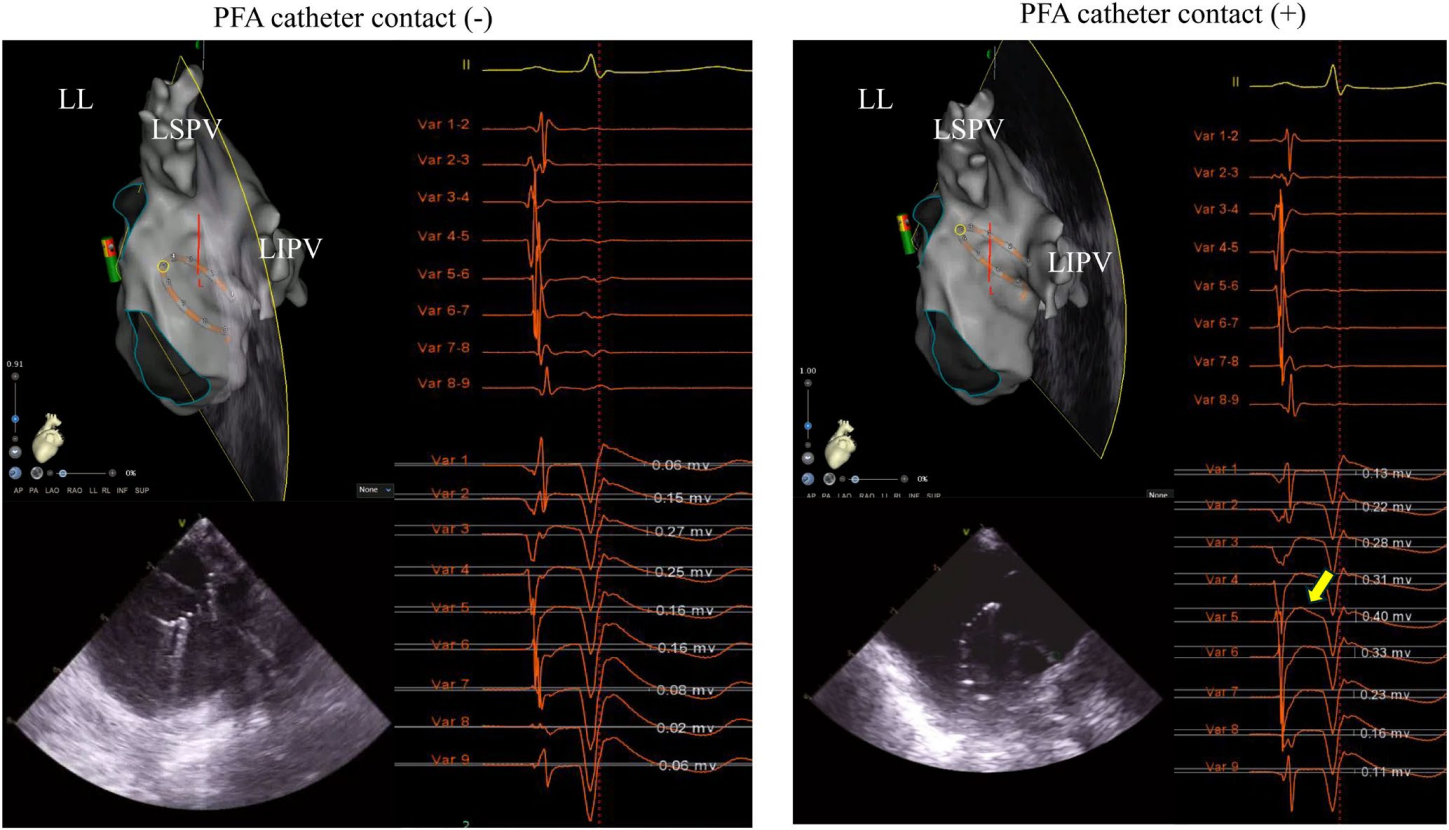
Myocardial contact of the pulsed field ablation (PFA) catheter was assessed using intracardiac echocardiography (ICE) and 3D electroanatomical mapping. In the absence of contact, both bipolar and unipolar recordings showed local potentials, but post‐P‐wave positive deflections were minimal. In contrast, when contact was confirmed, no significant change was observed in local electrograms, yet a marked increase in post‐P‐wave positive deflection was evident (yellow arrows). The contact site, located at the left pulmonary vein ridge, is indicated by yellow dots.
